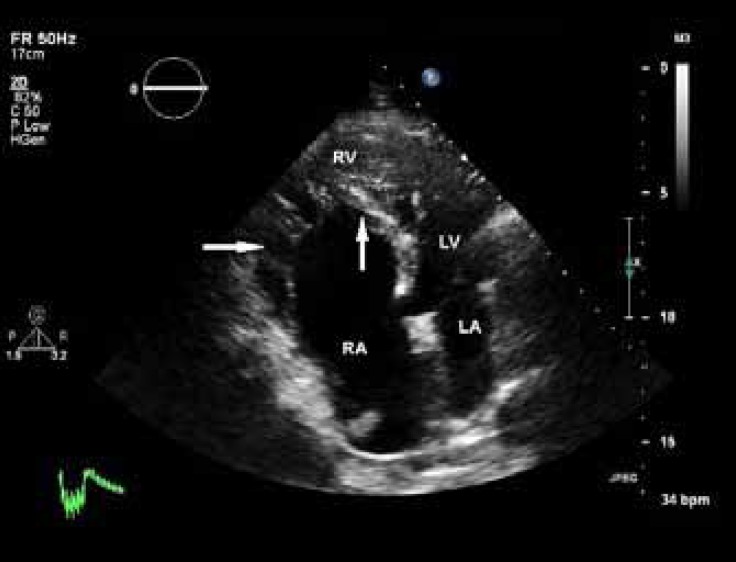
Apical four-chamber view in transthoracic echocardiography, showing an enlarged and pliable anterior tricuspid leaflet with mild tethering (horizontal arrow) and septal tricuspid leaflet with significant apical displacement (vertical arrow)
